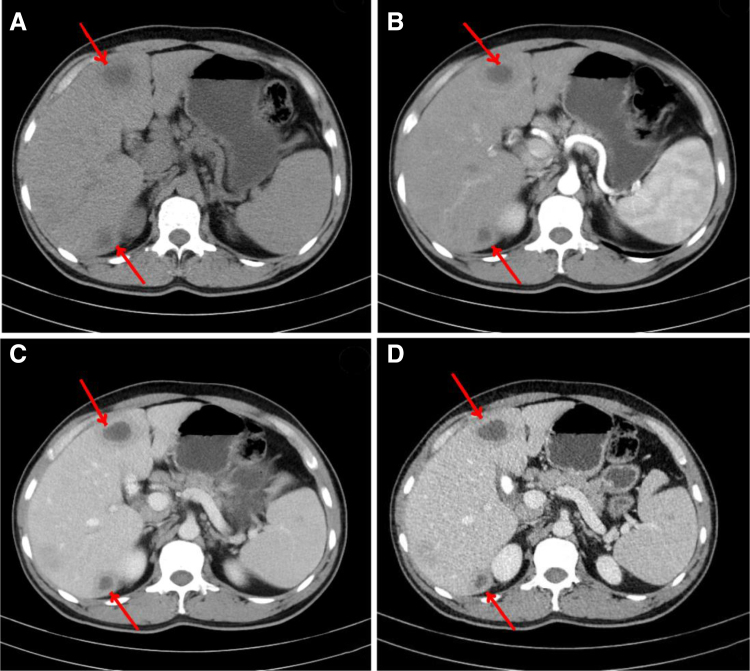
Contrast-enhanced abdominal CT: (A) Plain CT scan revealed multiple ring-shaped lesions with densities lower than that of the liver parenchyma and clear margins. (B) During the arterial phase, the lesions exhibited ring-like hypoenhancement. (C and D) In the equilibrium phase, contrast agent washout was observed. Red arrows indicate the location of the lesions. CT = computed tomography.
